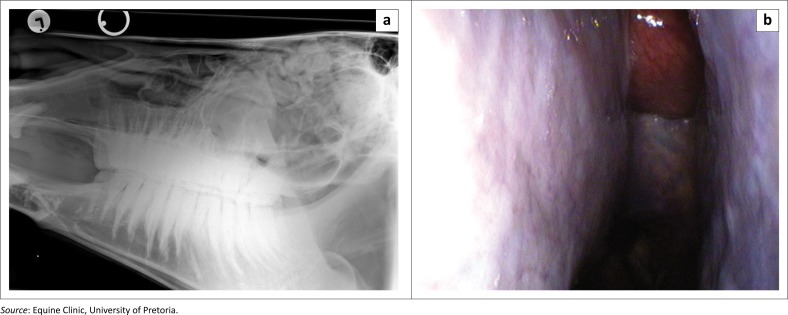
Initial ancillary diagnostic examinations of a 13-year-old Thoroughbred gelding presented with a bilateral nasal discharge. (a) Lateral head radiographs. Note the well-circumscribed soft tissue masses situated in the dorsal conchal sinuses and the fluid lines present within the caudal maxillary sinuses. Additional soft tissue opacities are visible in the ethmoidal region. (b) Endoscopic view of the left middle meatus. Note the red round mass extending from the left ethmoid region rostrally into the nasal cavity.
